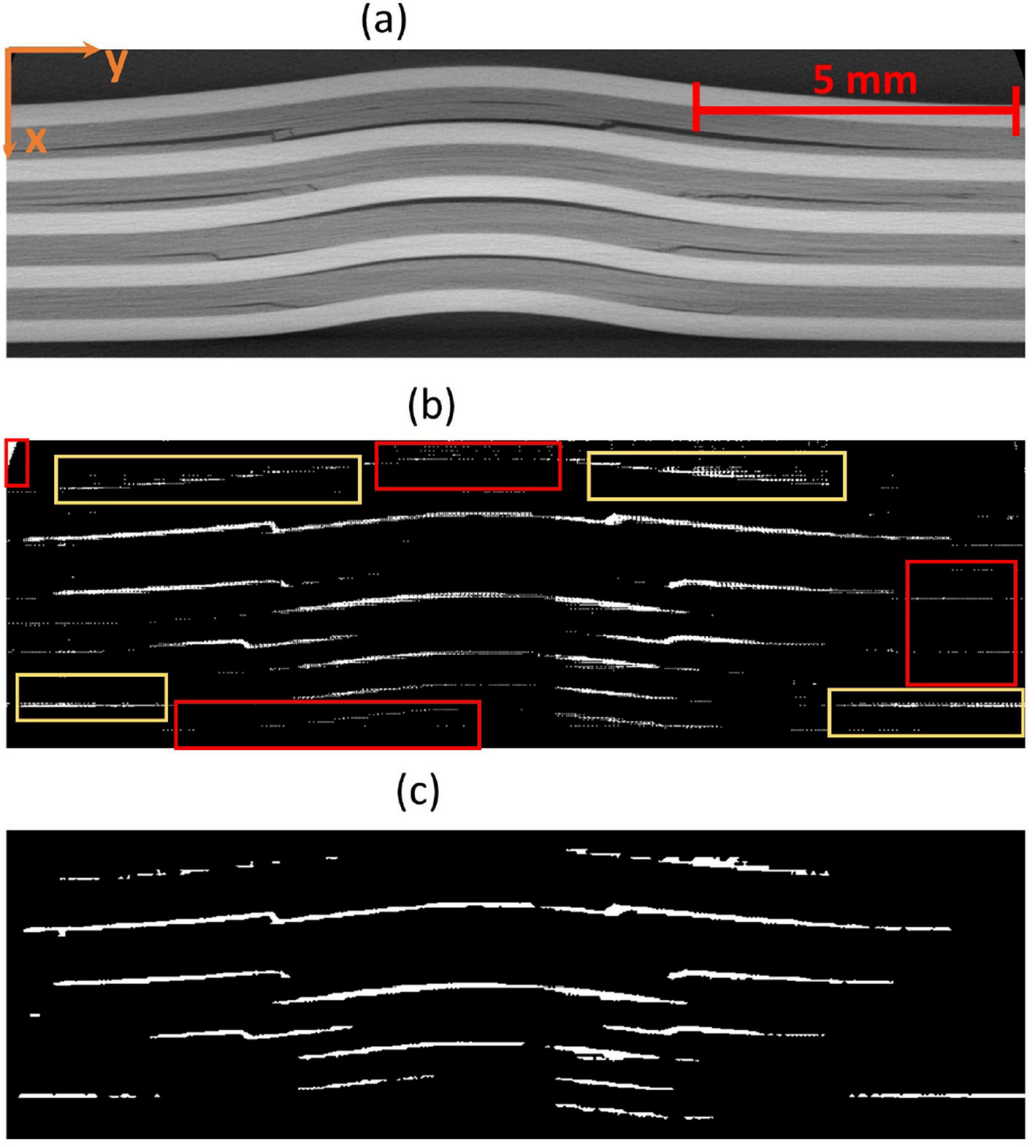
(a) An example CT slice of energy level 7.5J. (b) The mask produced by SAM generates a lot of false positives some examples are marked by red boxes. (c) The filter removes many of those false positives but still, some could not be removed as marked by the yellow box.
